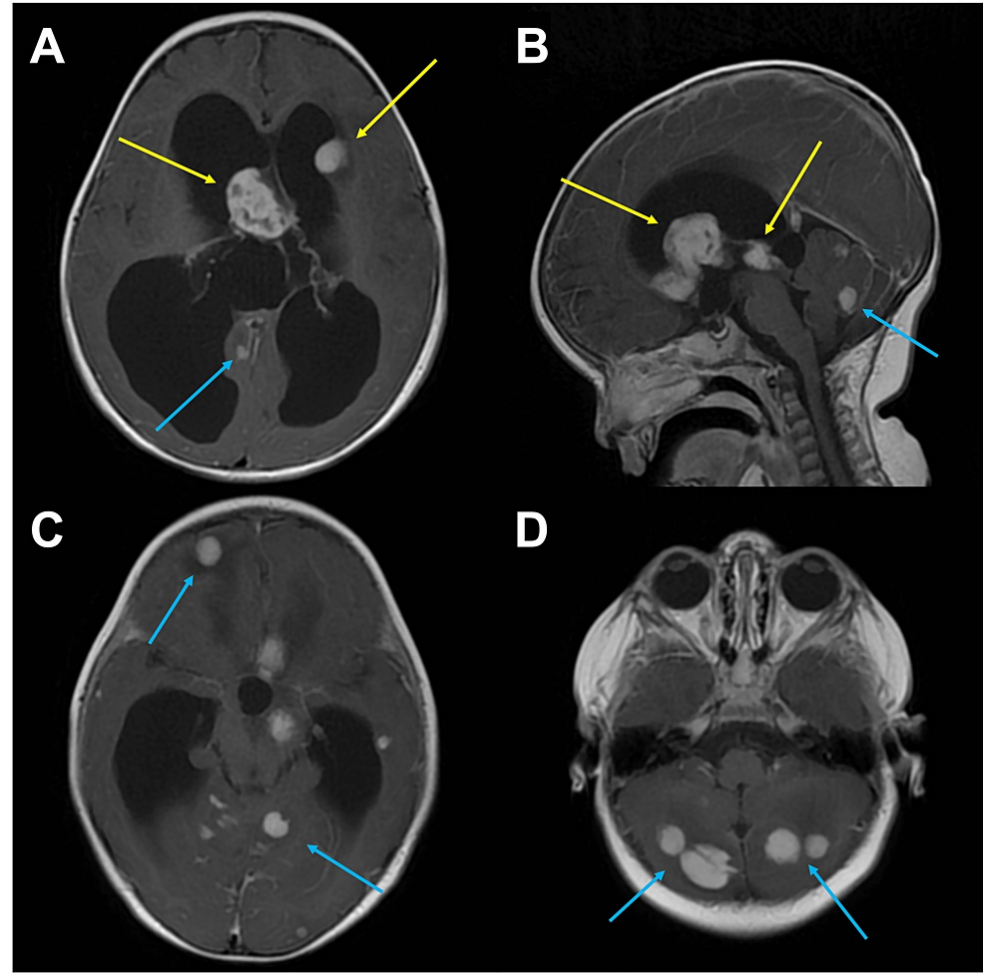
Initial Brain MRI at one year of age The axial (A) and sagittal (B) contrast-enhanced T1-weighted images depict lesions arising from the ventricular lining (yellow arrows), causing noncommunicating hydrocephalus due to the obstruction of the aqueduct of Sylvius. In addition, two additional axial images (C and D), also contrast-enhanced T1-weighted, reveal multiple enhancing brain parenchymal lesions within the supratentorial and infratentorial compartments (indicated by blue arrows).
